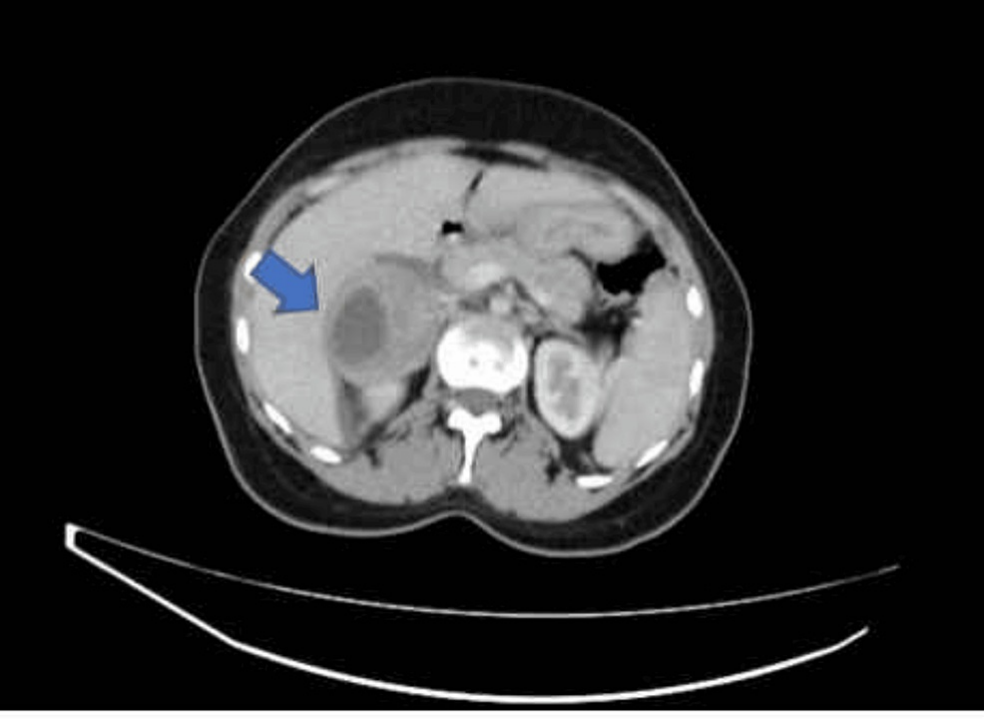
CT abdomen and pelvis with contrast showing right-sided adrenal mass.
